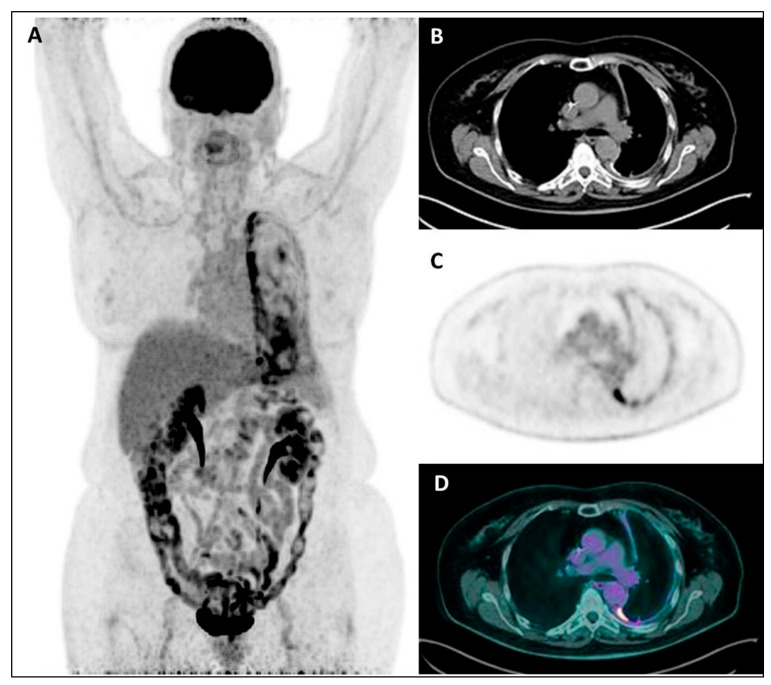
MIP (A), coregistered low-dose CT (B), ^18^F-FDG PET (C), and fused PET/CT images (D) in a female patient with recent diagnosis of MPM and submitted to talc pleurodesis: a diffuse increased^18^F-FDG uptake is evident throughout the left pleura, mainly along its mediastinal/para-vertebral side, with the CT evidence of a diffuse pleural thickening, sometimes with hyperdense spots due to talc collection.
